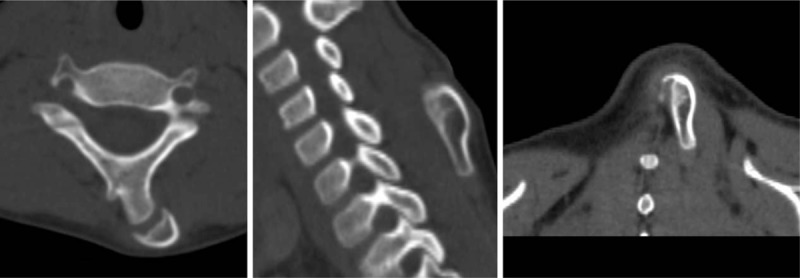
The reconstructed CT scans showed the well corticated anomalous bone articulating with the spinous process of C5. CT = computed tomography.
